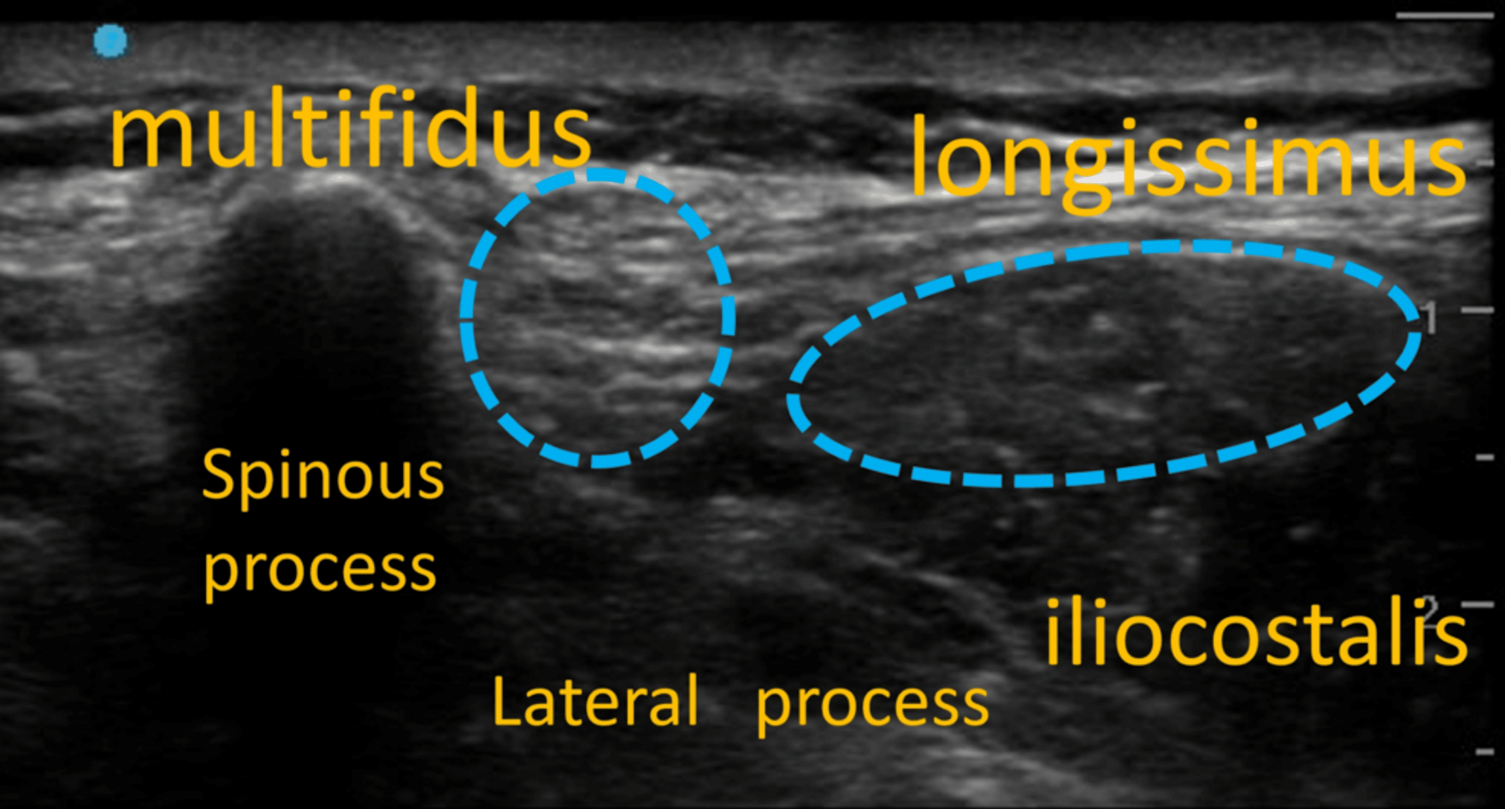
L4 ultrasound image before administering the TLIPB TLIPB, thoracolumbar interfascial plane block
